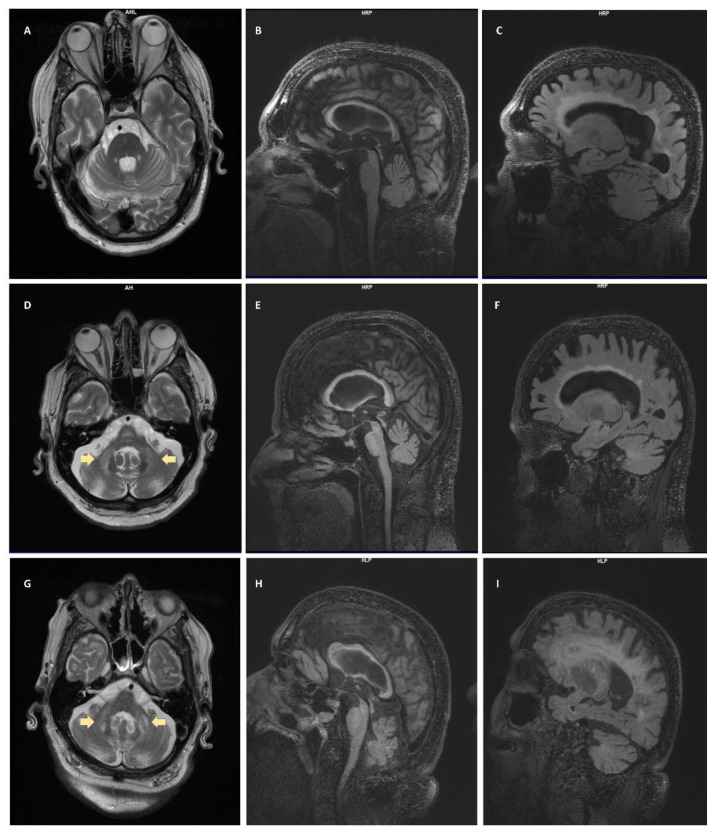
Radiological Evaluation. Case 1 (A) T2- weighted turbo spin echo (T2-TSE) no middle cerebellar peduncle (MCP) sign; (B) T2-weighted-Fluid-Attenuated Inversion Recovery (T2-FLAIR) splenium and genu signs; (C) T2-FLAIR general atrophy, ventriculomegaly. Case 2 (D) T2-TSE MCP sign (arrows); (E) T2-FLAIR splenium and genu signs; (F) T2-FLAIR general atrophy, ventriculomegaly, periventricular white matter disease (WMD). Case 3 (G) T2-TSE MCP sign (arrows); (H) T2-FLAIR splenium and genu signs; (I) T2-FLAIR general atrophy, ventriculomegaly, periventricular WMD.
